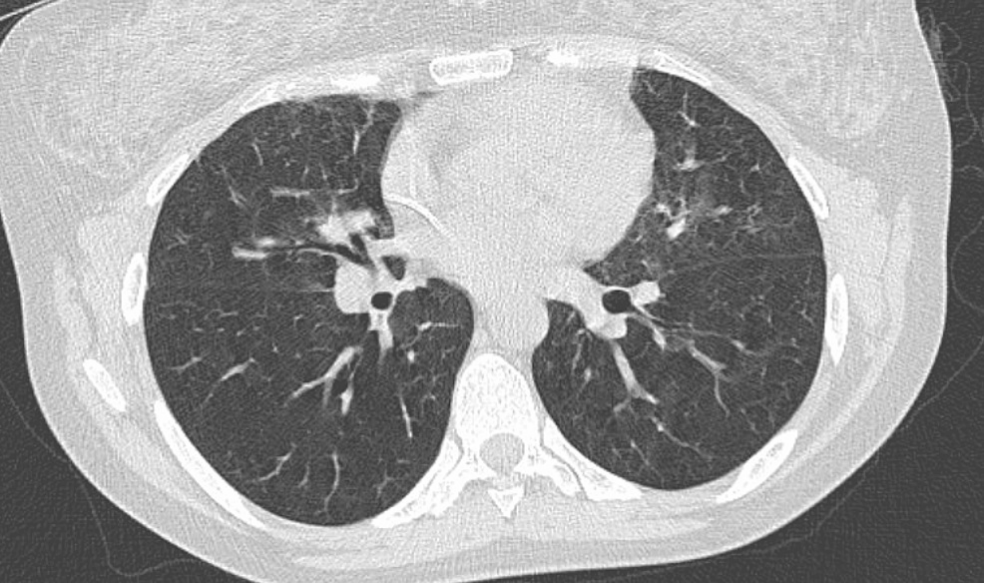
High-resolution chest computed tomography three months after starting rituximab and azathioprine showing significant interval decrease in mid-lower lung lobe patchy ground-glass opacities.
